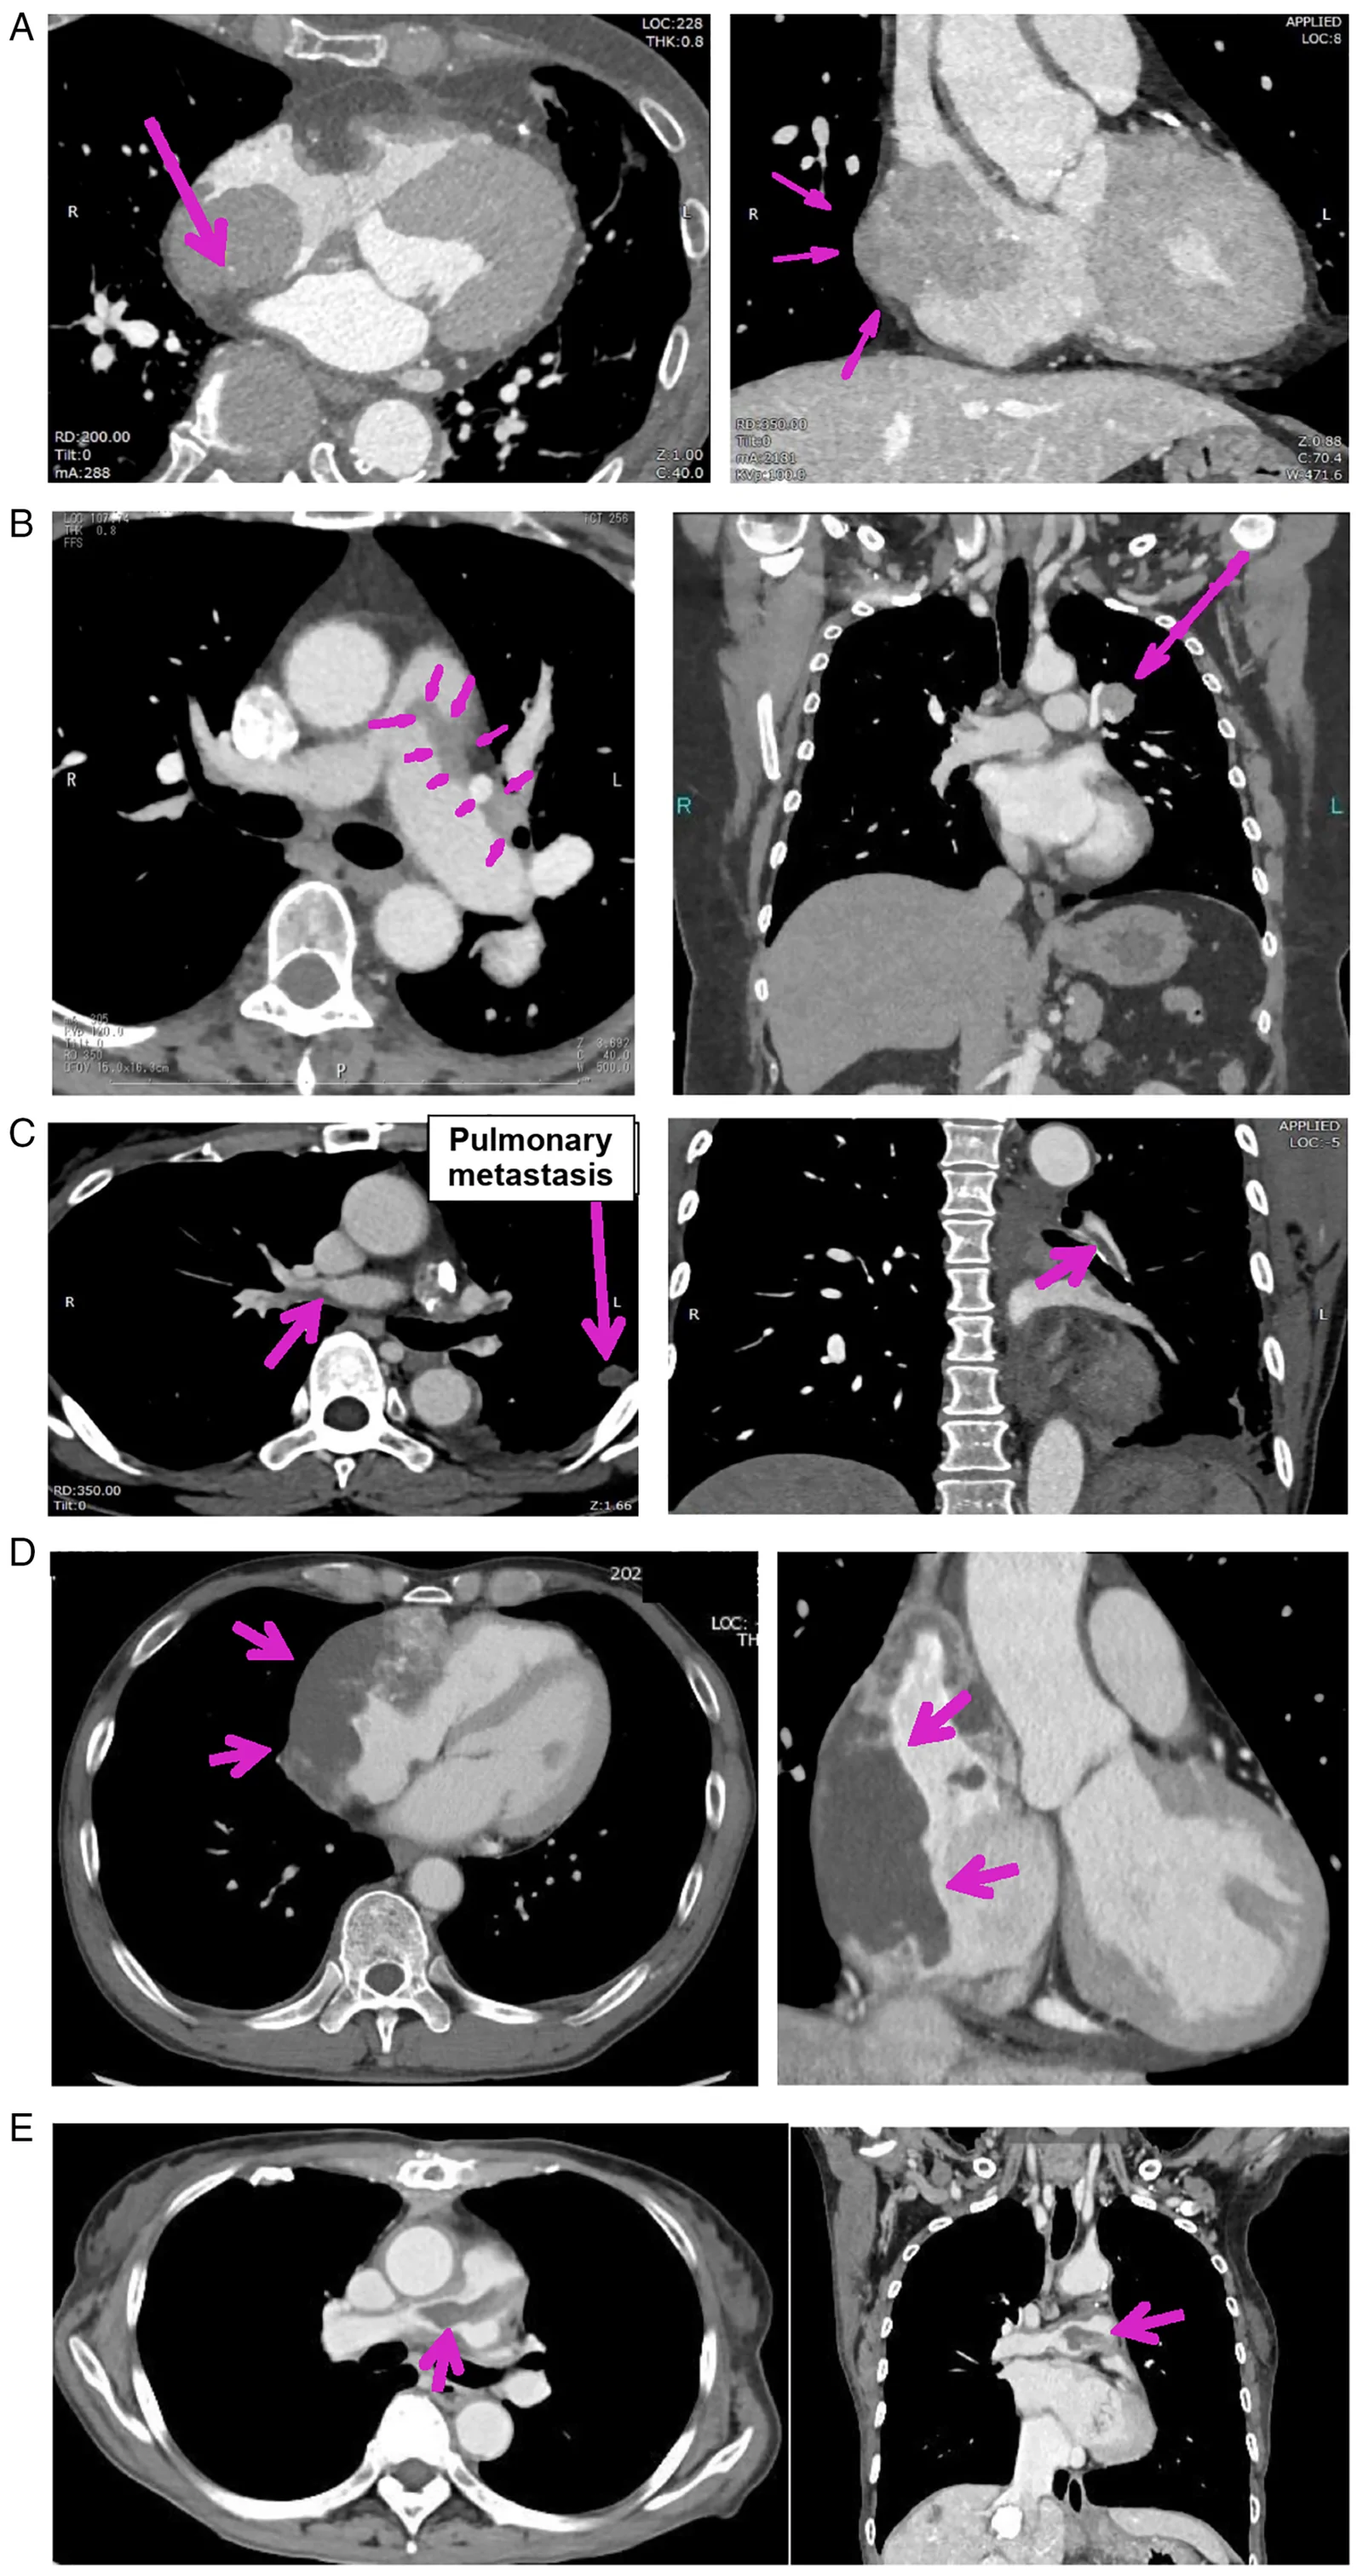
Contrast-enhanced computed tomography imaging. Arrows indicate the primary cardiovascular sarcoma lesion. (A) Case 1: Atrial angiosarcoma (75-year-old man). (B) Case 2: Pulmonary artery sarcoma (intimal sarcoma) (68-year-old woman). (C) Case 3: Pulmonary artery sarcoma (intimal sarcoma) (56-year-old woman). (D) Case 4: Atrial leiomyosarcoma (70-year-old man). (E) Case 5: Leiomyosarcoma (81-year-old woman).
